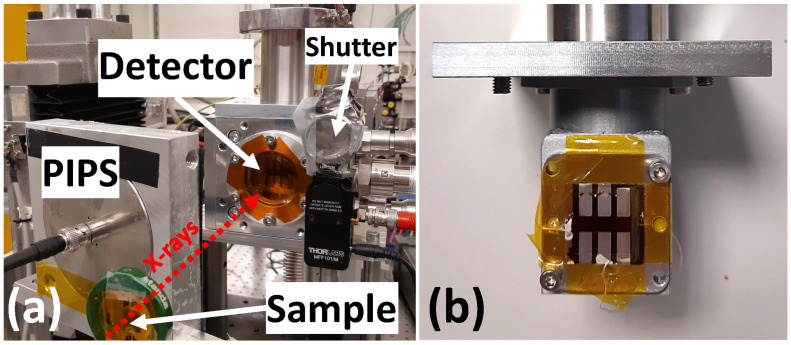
(a) Experimental setup at the PETRA-III P64 beamline. The hybrid detector, shutter, sample, and PIPS diode are visible. The direction of the incoming X-rays is indicated. (b) Enlarged view of the hybrid detector. Six independent pixels with aluminum contacts are visible.
